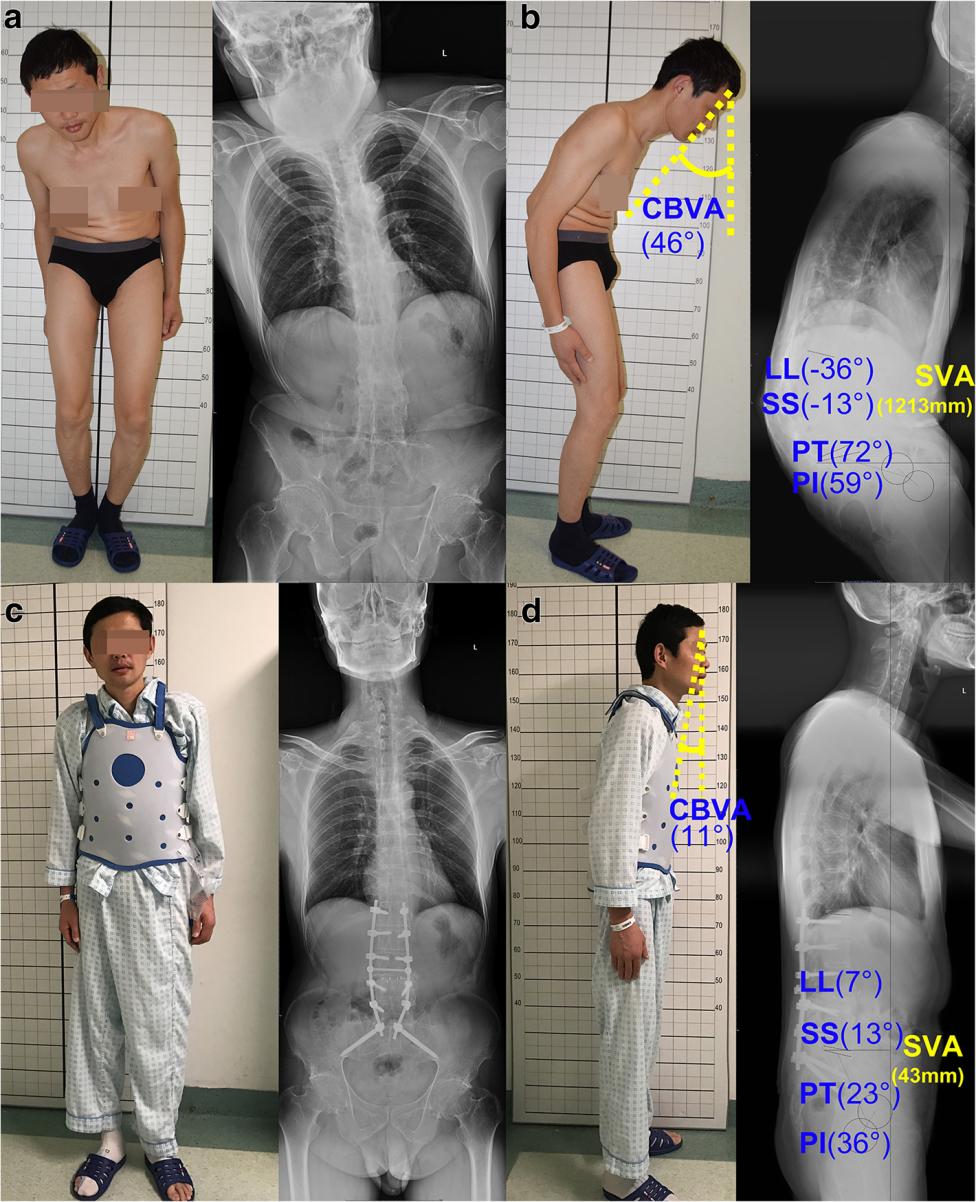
An example patient (46-year-old male) presents the severe kyphosis deformity. The most improvement of the SRS activity appears 1.5 pre-operation and 4.8 post-operation. The postoperative satisfaction with management score is 5.0. The SRS Appearance score increases from 2.0 to 4.0, the SRS pain score increases from 3.6 to 4.4, and the SRS mental health score increases from 2.8 to 4.2. Preoperative a posteroanterior and b lateral radiographs and postoperative c posteroanterior and d lateral radiographs
